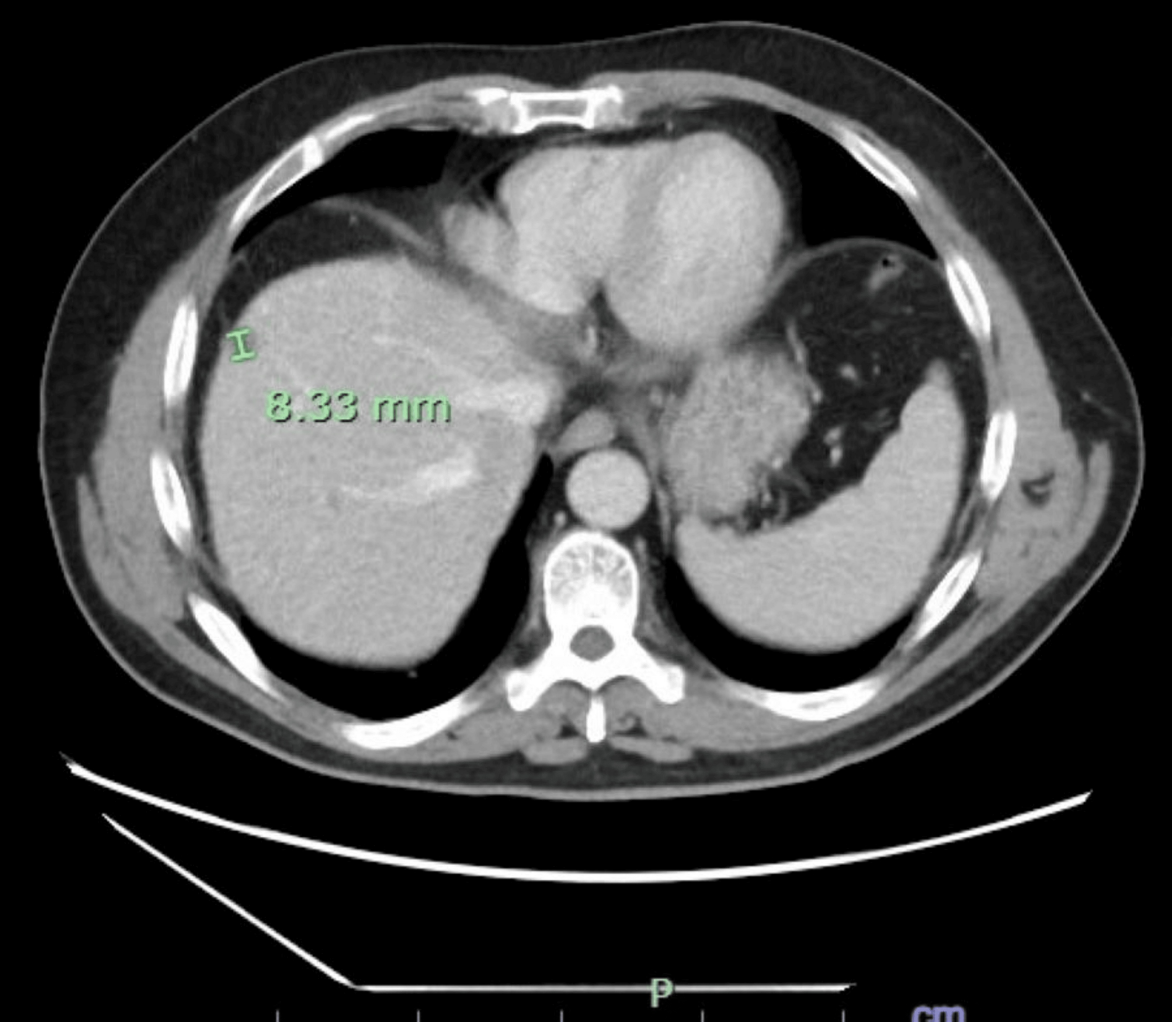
Additional abdominal CT slice demonstrating a third hepatic lesion and nodular liver contour.
